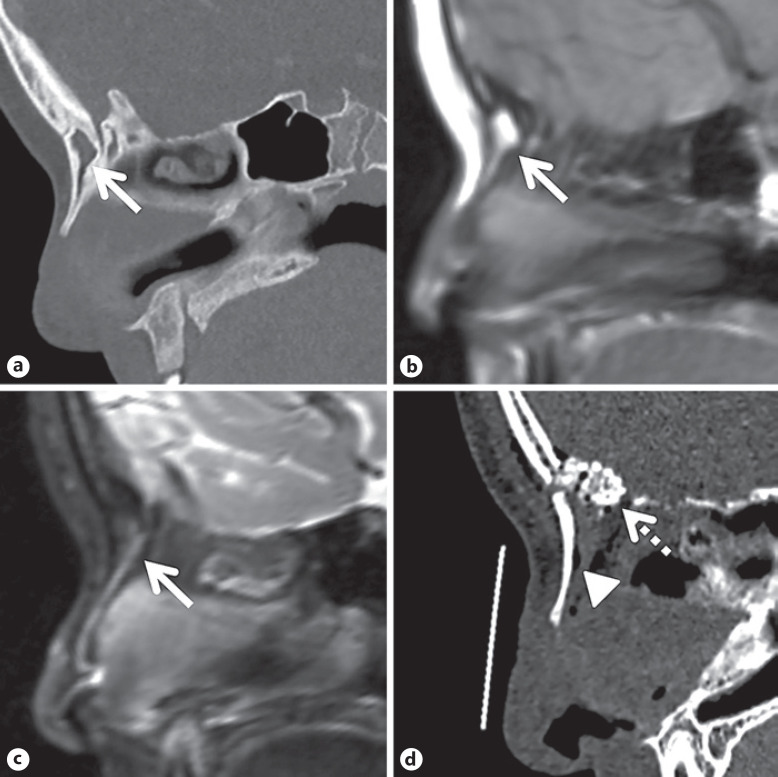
a Sagittal head CT showing intraosseous cystic lesion completely hidden in the nasal bone. b T1-weighted brain magnetic resonance imaging showed hyperintense cystic contents. c Short tau inversion recovery sequence demonstrated fat-suppressed signals in the corresponding area. d Post-operative head CT demonstrating replaced nasal bone (arrowhead) and particulate corticocancellous bone (dashed arrow) reconstructing the skull base defect.
